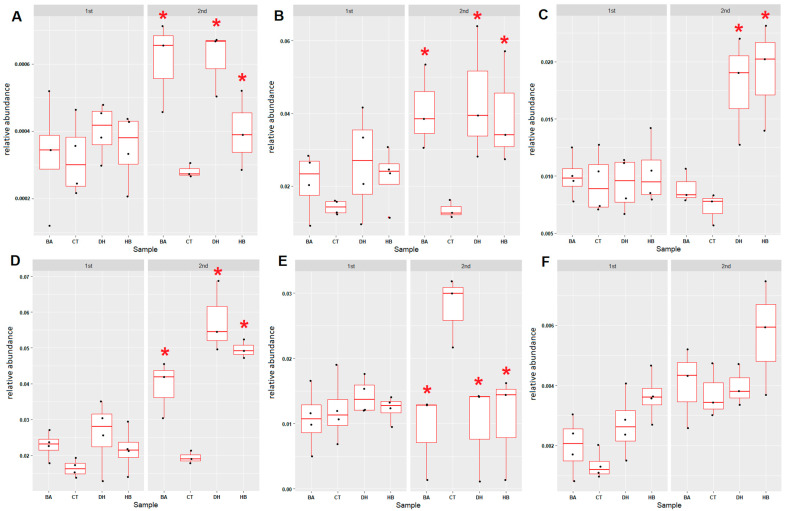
Overall relative abundance changes in nitrogen-cycle-associated microbial communities in the BA-, HB- and DHA-treated river sediments. (A) Anammox (anaerobic ammonium oxidation). (B) Nitrogen fixation (M00175: nitrogen => ammonia). (C) Denitrification (M00529: nitrate => nitrogen). (D) Dissimilatory nitrate reduction (M00530: nitrate => ammonia). (E) Nitrification (M00528: ammonia => nitrite). (F) Assimilatory nitrate reduction (M00531: nitrate => ammonia). The term “1st” indicates the period between the 0th week to the 8th week; “2nd” indicates the period between the 8th week to the 17th week. Red stars indicate the *p*-value of the Mann–Whitney U test < 0.05 (compared with CT). CT: control; BA: benzoic acid; HB: 4-hydroxybenzoate; DH: dehydroacetic acid.
